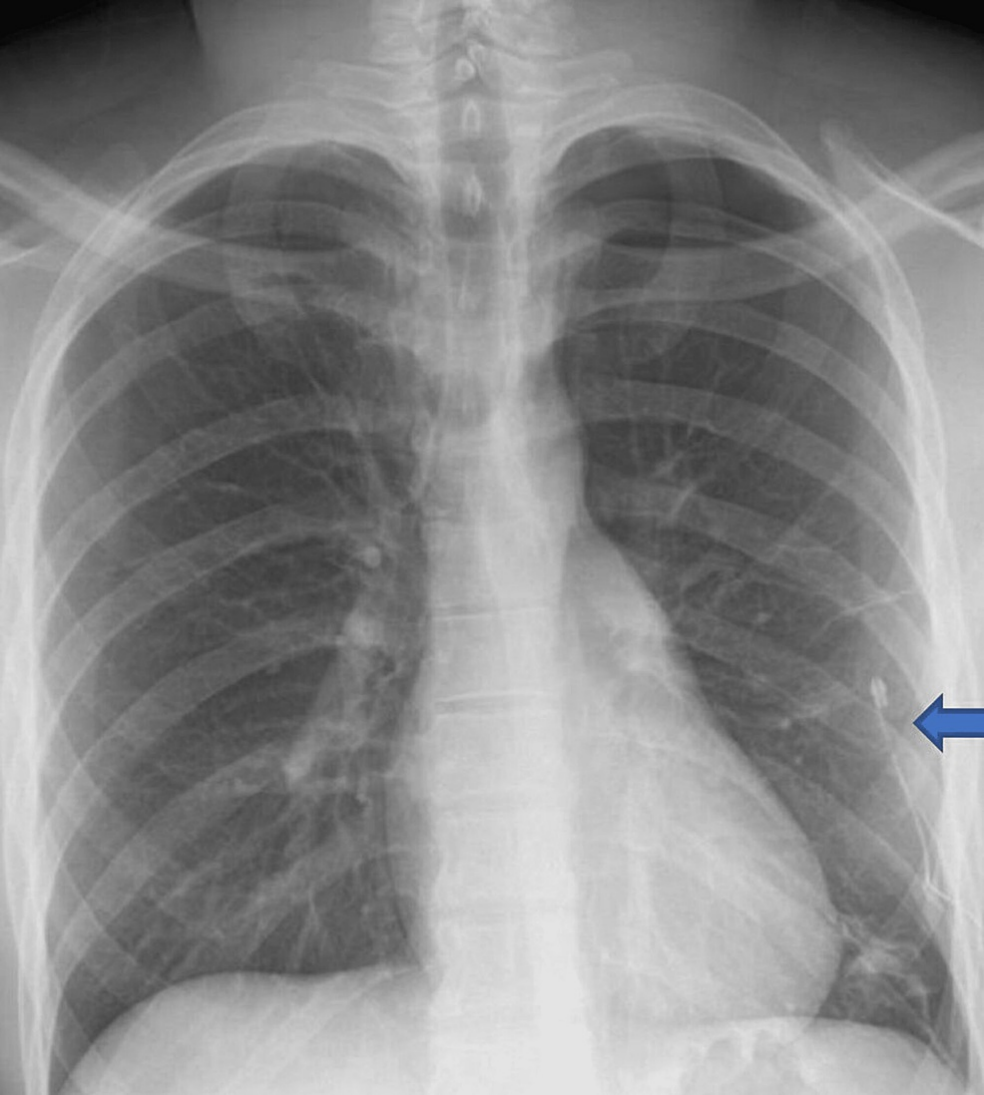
Chest radiography post drain insertion showing complete resolution of the left-sided pneumothorax and chest drain tip shown by the arrow.
